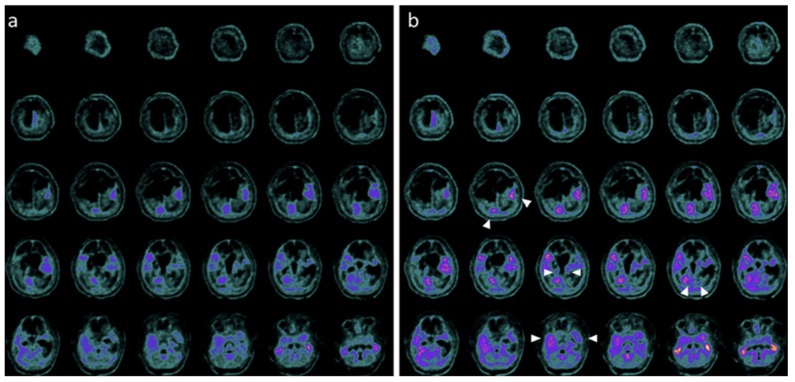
PET before and after the treatment with hr-NGF in the second TBI patient. (a,b) Brain 18F-FDG PET axial slices performed before (a) and after (b) hr-NGF treatment. A severe global reduction in 18F-FDG uptake was observed in all cortical and subcortical regions as well as in the cerebellum (a). After hr-NGF administration, an increase in radiotracer uptake was detected in the left and right temporal cortex (right: +18%; left: +15%), bilateral parietal cortex (right: +18%; left: +15%), right and left occipital cortex (right: +10%; left: +13%), and right and left thalamus (+7% and +8%, respectively). The white arrows delineate the aforementioned areas. (b).
